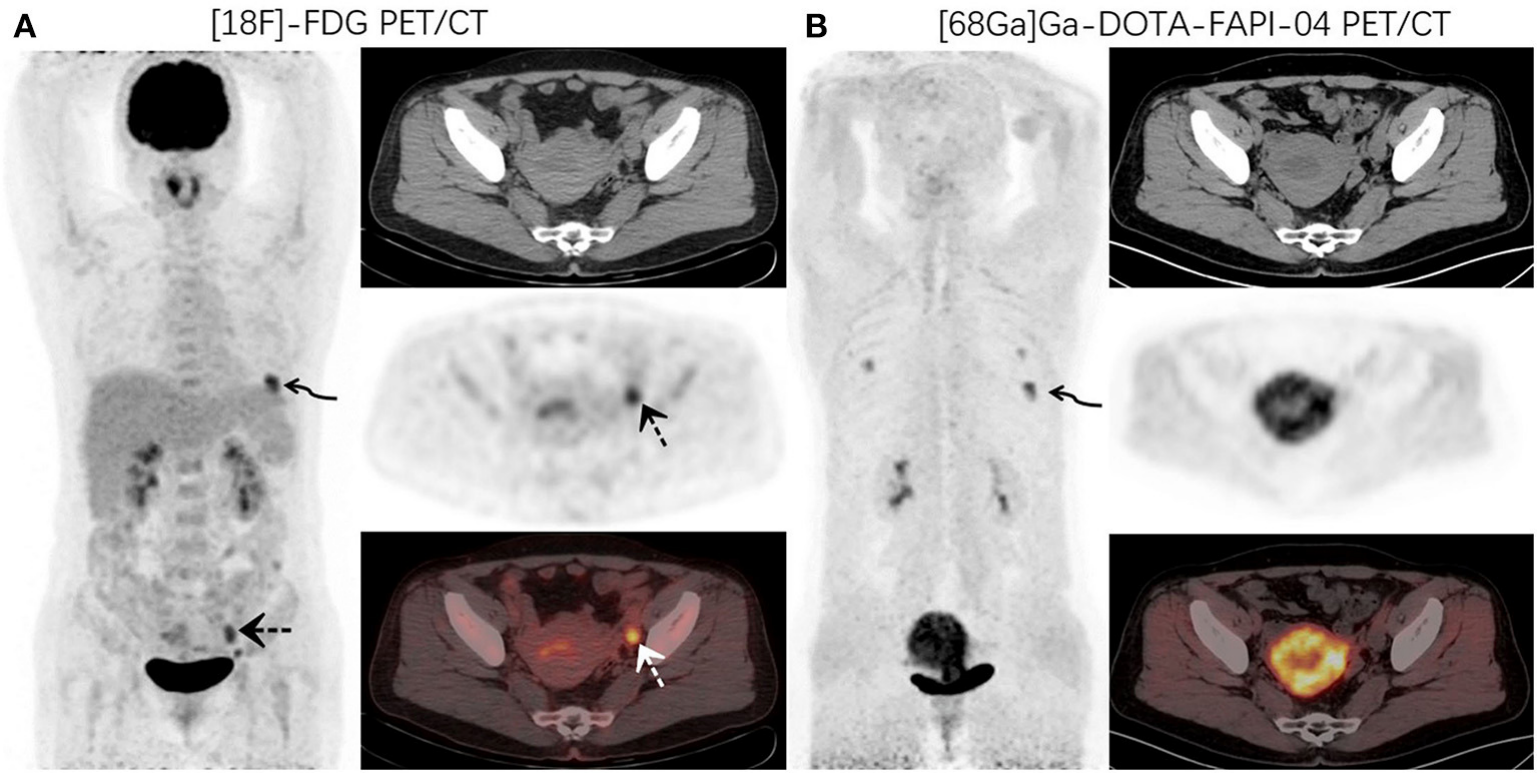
A 48-year-old woman with newly diagnosed lung cancer underwent a PET/CT scan for tumor staging. ^18^F-FDG PET/CT (A) images revealed increased FDG uptake in the left lung (curved arrow, SUVmax9.1). Abnormal activity was also observed in the endometrium and left adnexal area (dotted arrow, SUVmax 5.2). ^68^Ga-DOTA-FAPI-04 PET/CT (B) showed increased FAPI uptake in the left lung lesion (curved arrow, SUVmax10.6) and uterus. No other abnormal lesions were observed.
